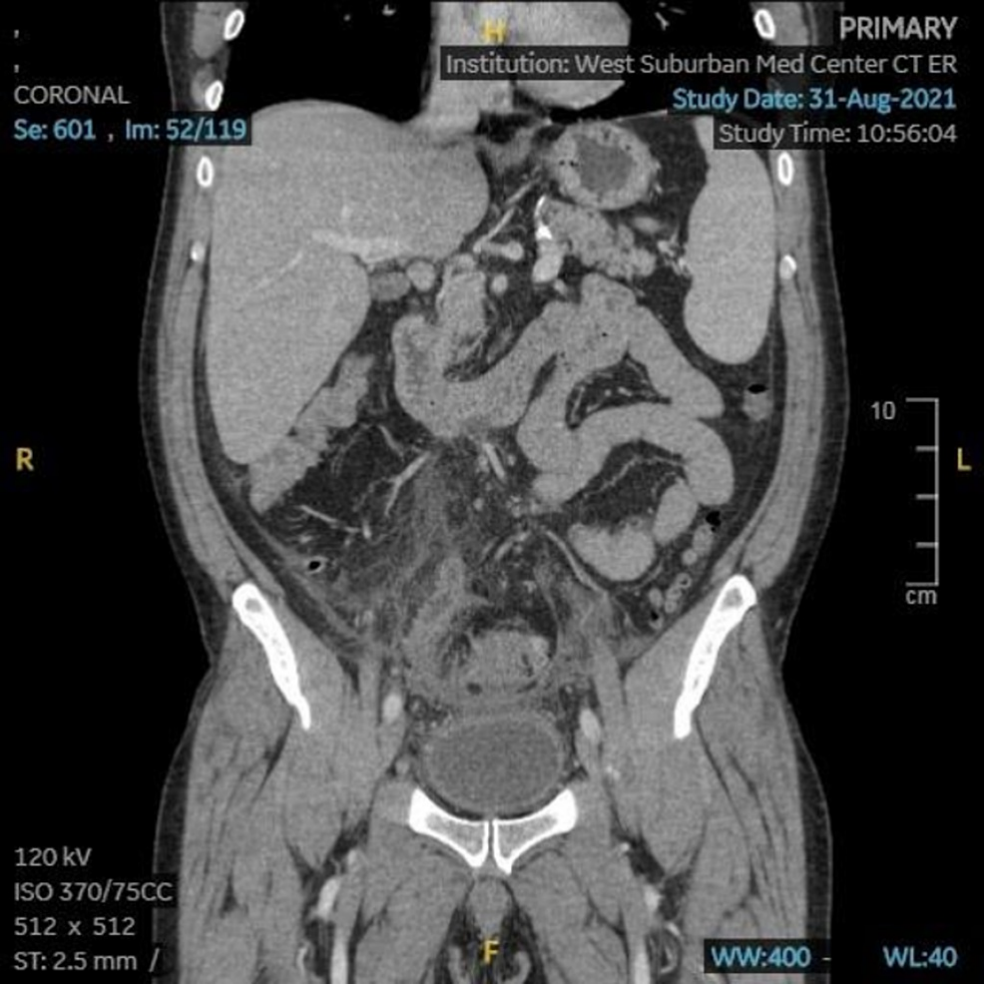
Abdominal CT (coronal) showing bowel wall thickening and small foci of gas next to the sigmoid colon suggestive of perforation.
